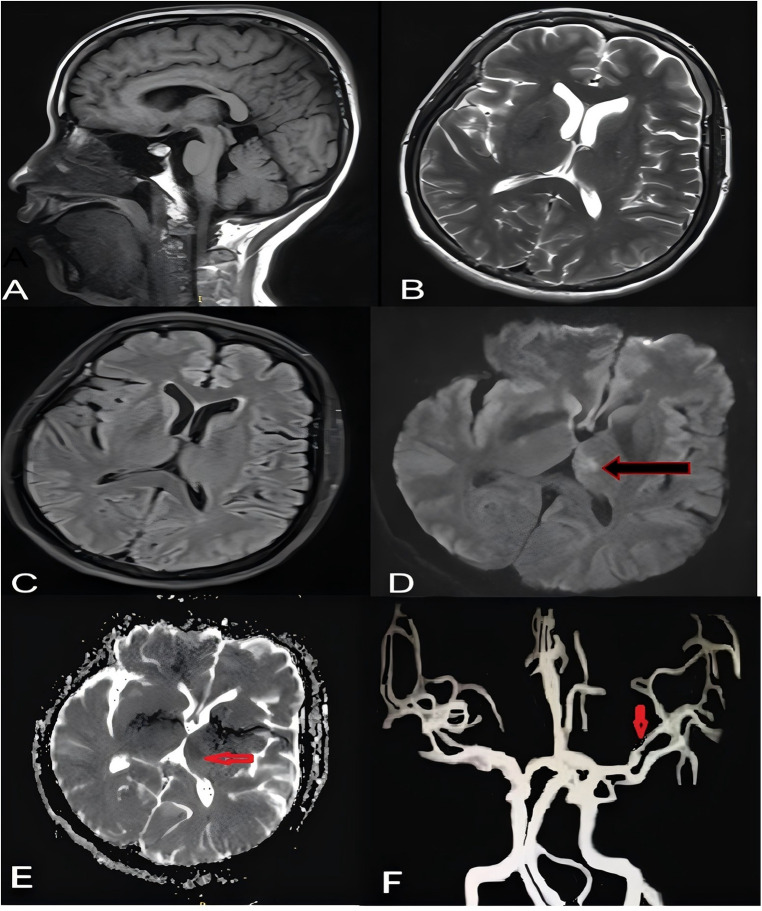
Brain magnetic resonance imaging performed 5 h after stroke onset. T1-weighted, T2-weighted, and fluid-attenuated inversion recovery images show no obvious abnormalities (A–C). Diffusion-weighted imaging demonstrates a hyperintense lesion in the left thalamus [(D), long black arrow] with corresponding hypointensity on the apparent diffusion coefficient map [(E), short red arrow], consistent with acute infarction. Magnetic resonance angiography reveals mild stenosis of the superior trunk of the left middle cerebral artery [(F), short red arrow].
